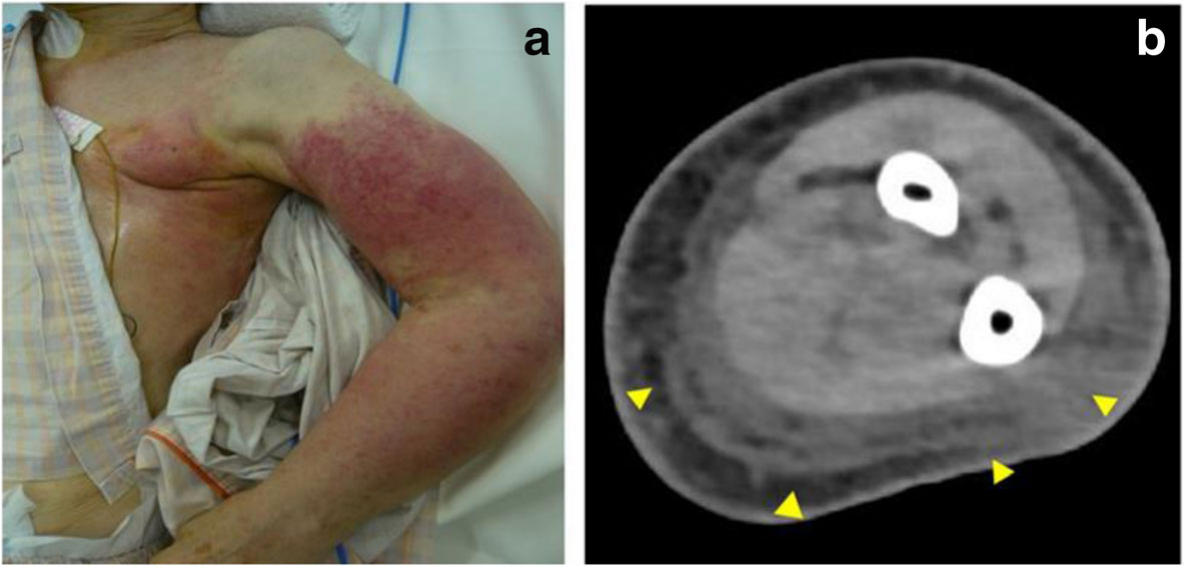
a Generalized erythematous macular rash on the left arm. b Computed tomography of the left arm, showing thickening of the superficial fascial layer (*triangles*)
